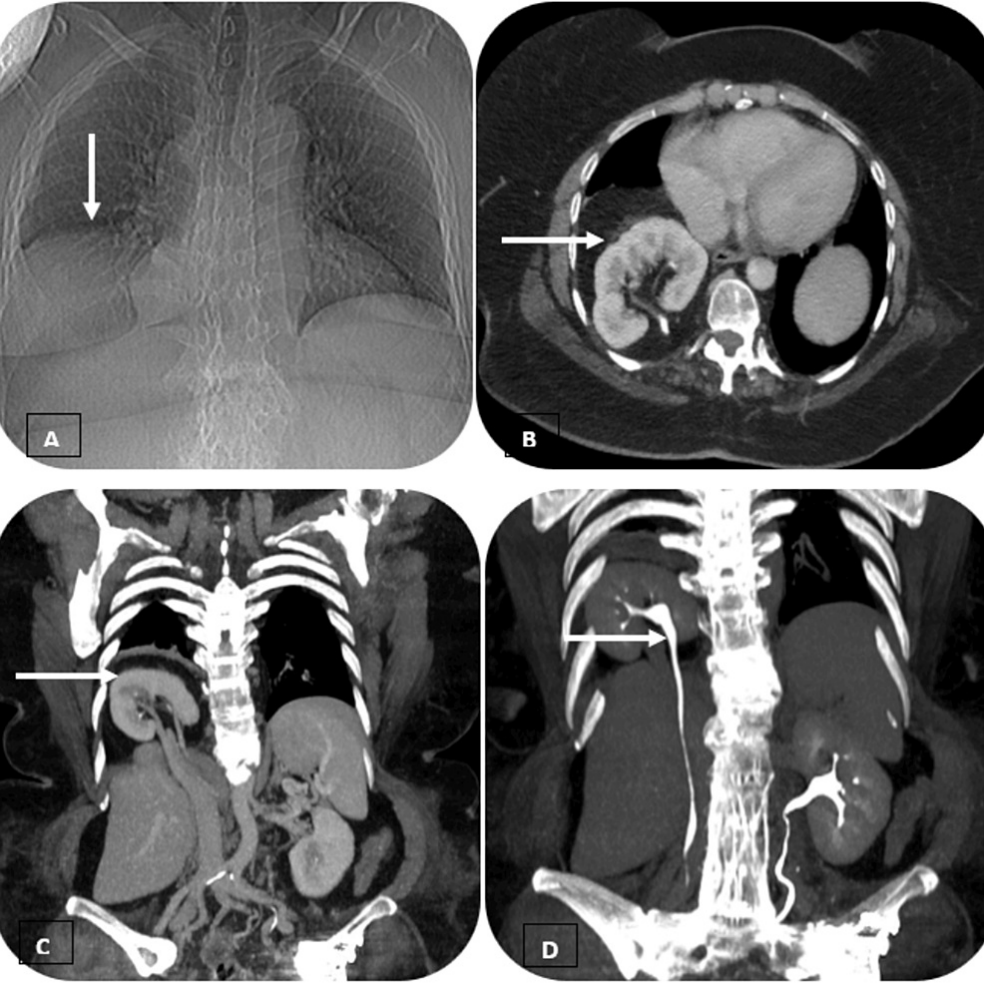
A: Radiograph of the chest showed elevated right dome of the diaphragm (white arrow); B (axial view) and C (coronal view): contrast-enhanced CT (CECT) of the chest and abdomen show normal size, attenuation and enhancement of the ectopic right intrathoracic kidney (white arrow); D: Coronal view of CT urography shows prompt excretion of contrast into the pelvicalyceal system of ectopic right intrathoracic kidney (white arrow).
